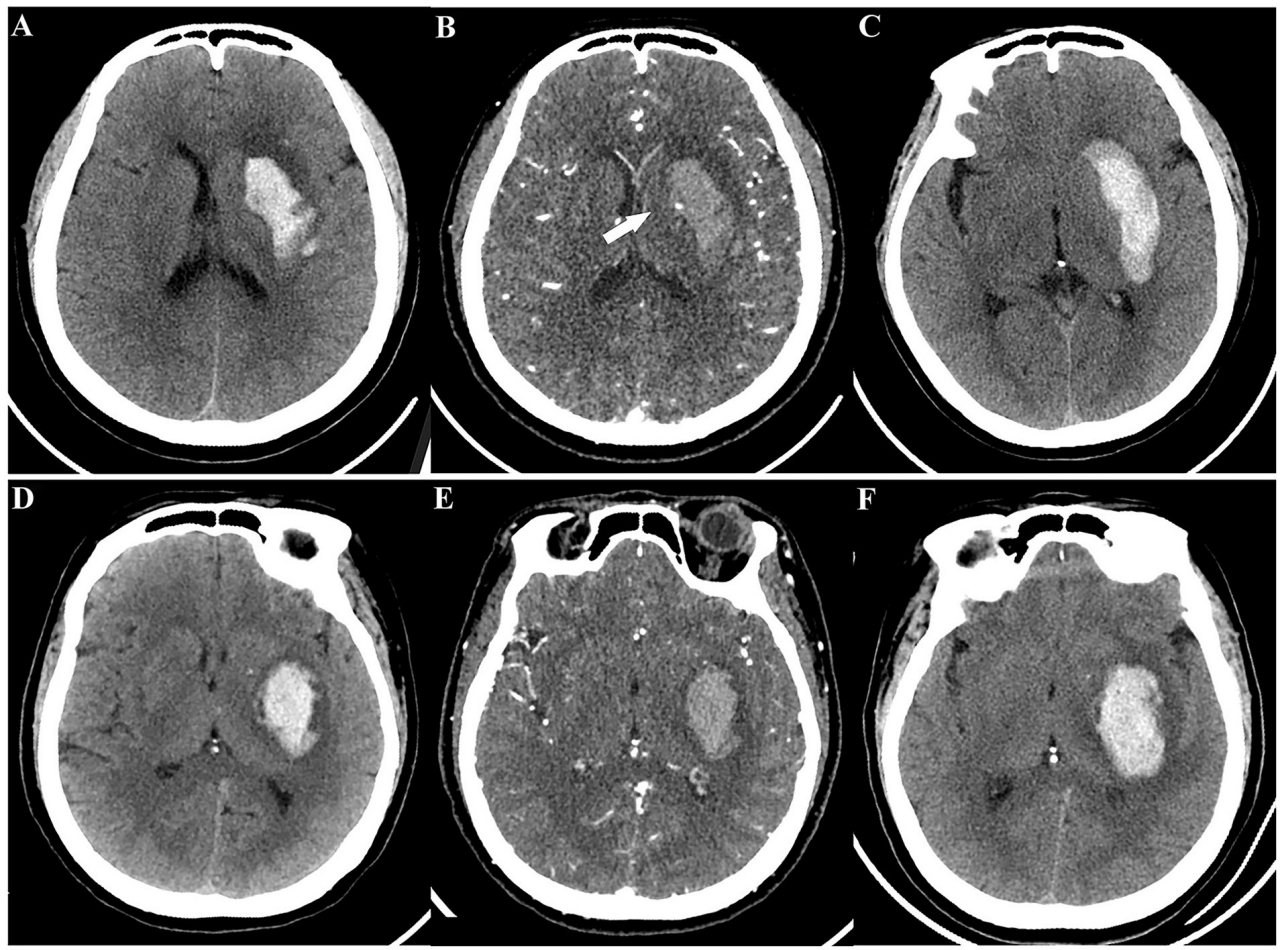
A comparison of two spontaneous intracerebral hemorrhage cases (with and without the spot sign) according to radiomics models. Case 1 with CTA spot sign, a 49-year-old woman: (A) initial NCCT; (B) CTA showed spot sign (white arrow); (C) follow-up CT revealed HE. Case 2 without CTA spot sign, a 55-year-old man: (D) initial NCCT; (E) CTA did not show spot sign; (F) follow-up CT still revealed HE. Both radiomics models provided successful predictions for these cases. HE, hematoma expansion; NCCT, non-contrast computed tomography; CTA, computed tomography angiography.
